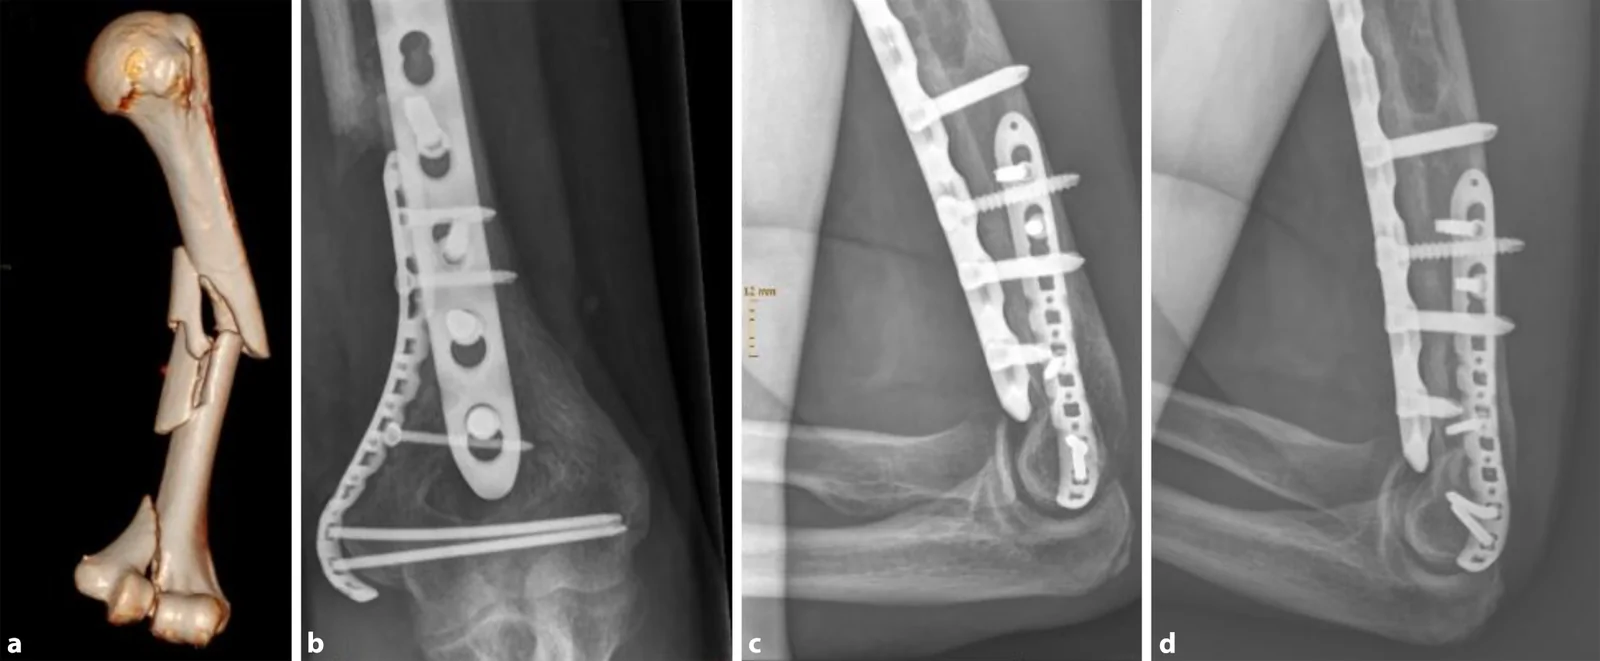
A 48-year-old man sustained a segmental humerus fracture with articular involvement. a Preoperative computed tomography. b, c X-rays obtained at the 3‑month follow-up with the flip osteotomy still visible. d After 1 year the flip osteotomy is fully healed
